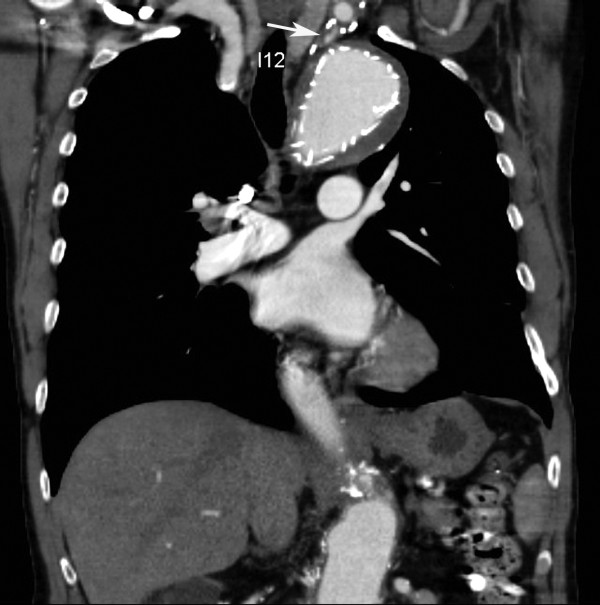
Coronal CT-reconstruction image 6 months after stent-grafting reveals a faint opacification (white arrow) of the proximal left subclavian artery with focal opacification of the aneurismal sac lumen.
